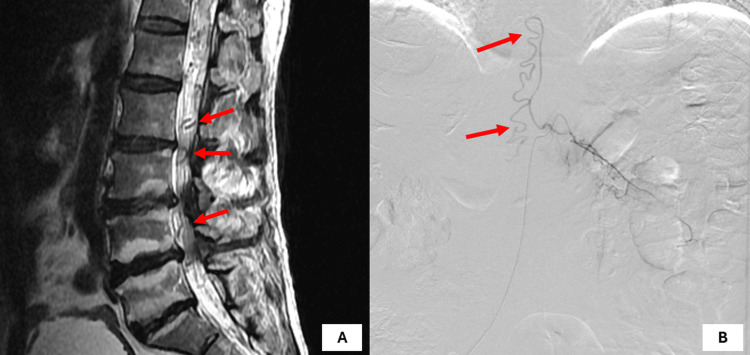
(A) Preoperative MRI and (B) DSA revealed AVM at L1 level (type 4A) AVM: arteriovenous malformation; DSA: digital subtraction angiography; MRI: magnetic resonance imaging
